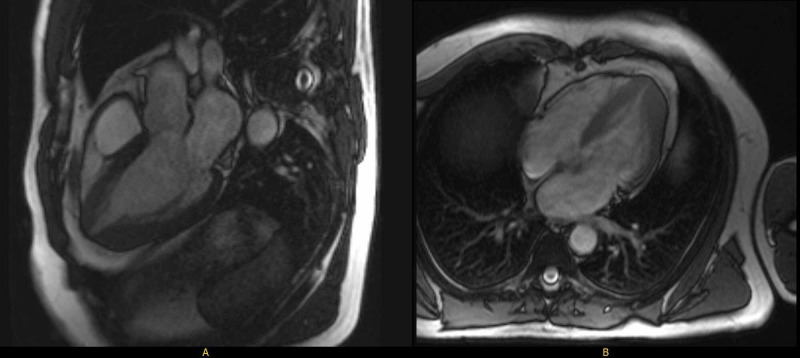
Cardiac MRI showing apical hypertrophy with 17 mm thickness of apex
